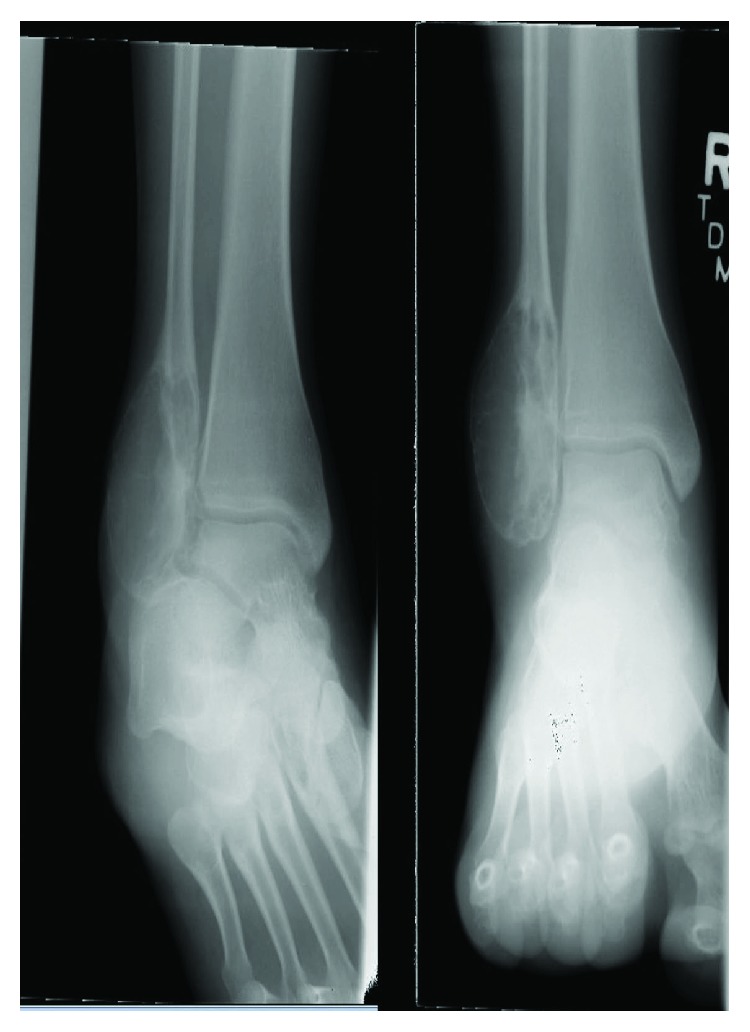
AP and mortise radiographs of the ankle demonstrating a lytic, expansile, geographic bone lesion with no cortical disruption.
